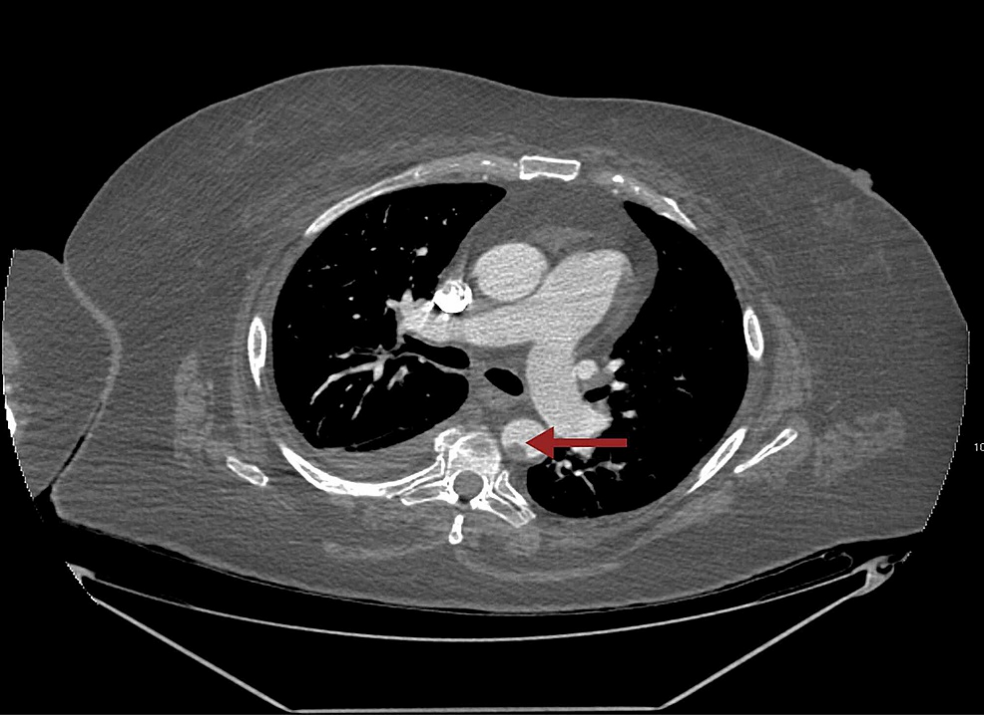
CT angiogram of the chest with the arrow pointing towards mural thrombus within the descending thoracic aorta.
